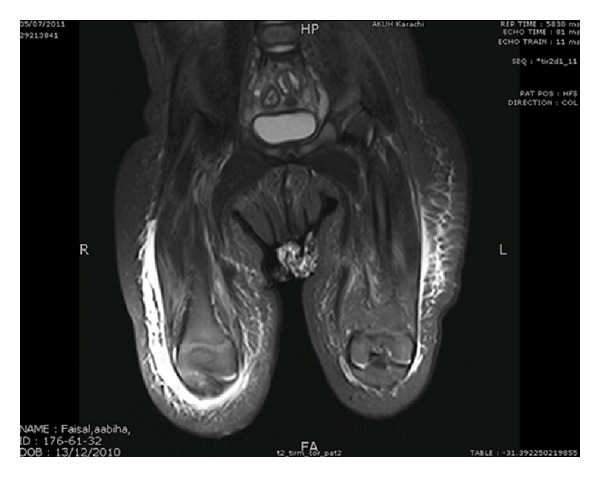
MRI showed extensive edema involving skin, subcutaneous tissue, and deep fascia with multiple areas of negative enhancement in the deep subcutaneous fascia representing small collections.
